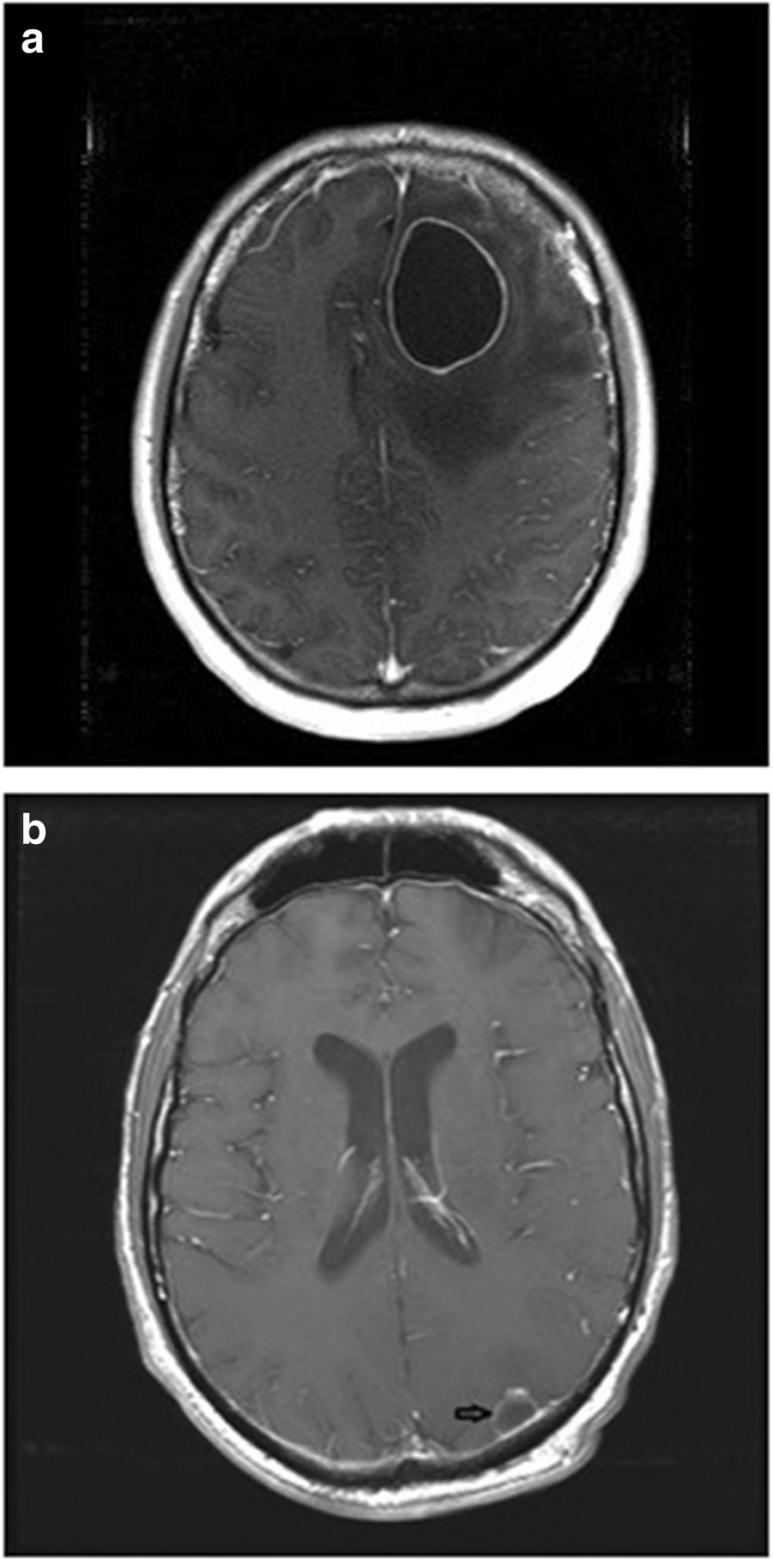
Patient 2 imaging. MRI showing (a) left frontal glioblastoma multiforme (GBM) and (b) subsequent left parietal recurrence (arrow).
